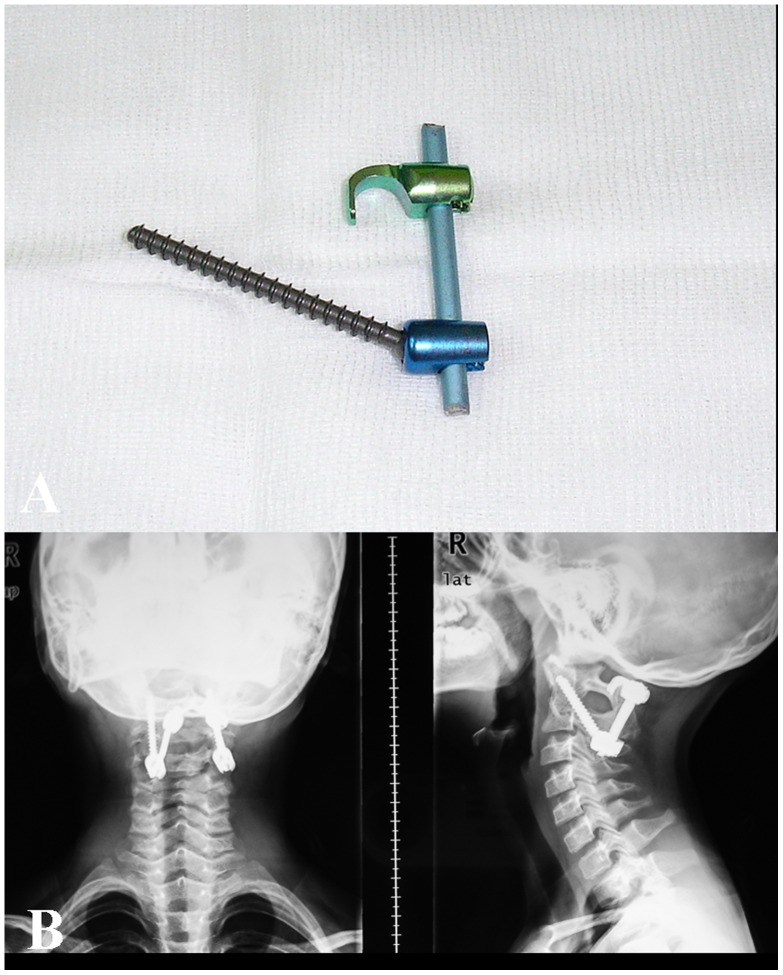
A modified implant and postoperative radiograph of patient 1. A color photograph (A) showing C1-2 transarticular hooks and C1 laminar hooks implant. Postoperative lateral and anteroposterior view radiographs (B) demonstrating the satisfactory position of implants.
